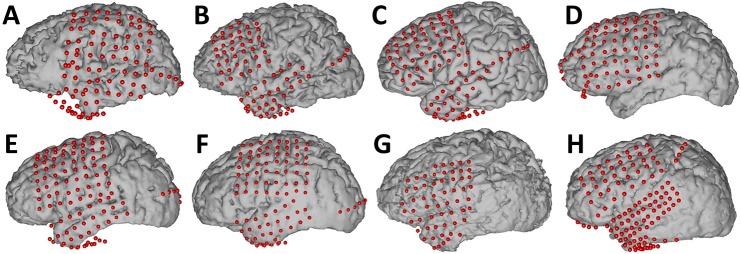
Placement of electrodes on all 8 subjects. Electrode locations were identified in a post-operative CT, co-registered to preoperative MRI, and transformed into a joint (Talairach) space for comparison across subjects. An average brain is shown for Subject C as a 3D rendering was not available.
